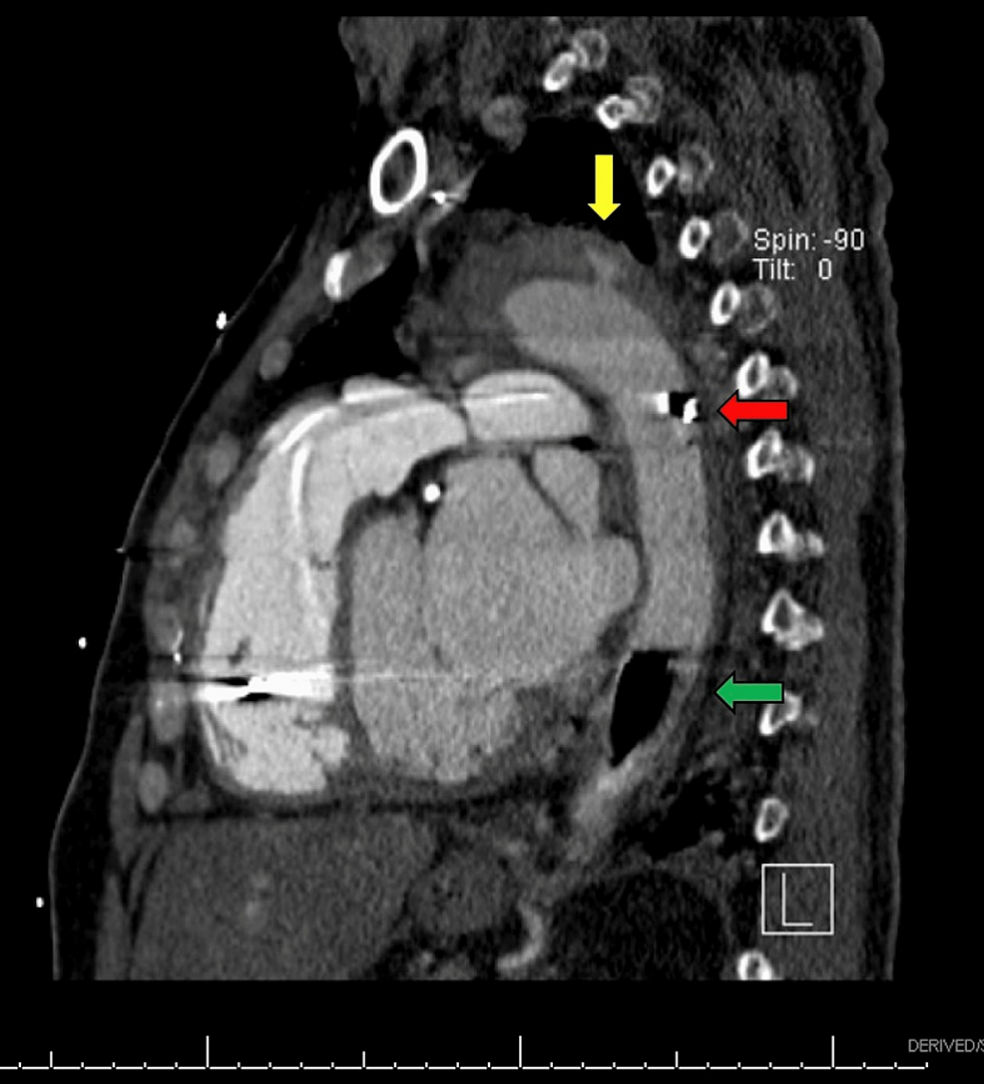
Parasagittal view of CT angiogram demonstrating a tear within the descending aorta with hematoma formation (yellow arrow). Intra-aortic balloon pump catheter tip (red arrow) and balloon (green arrow).
